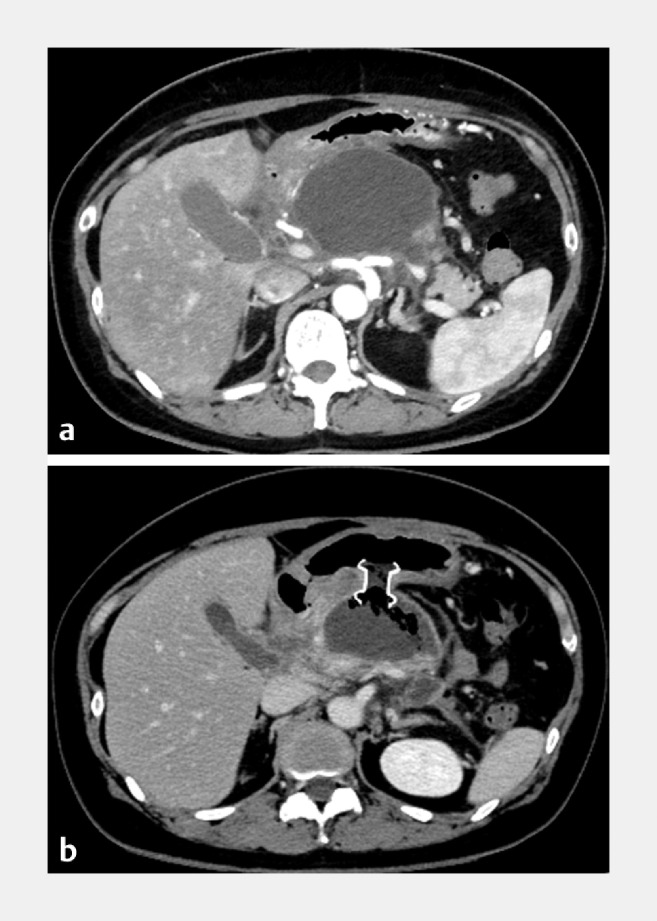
Dynamic computed tomography images of walled-off necrosis (WON).
